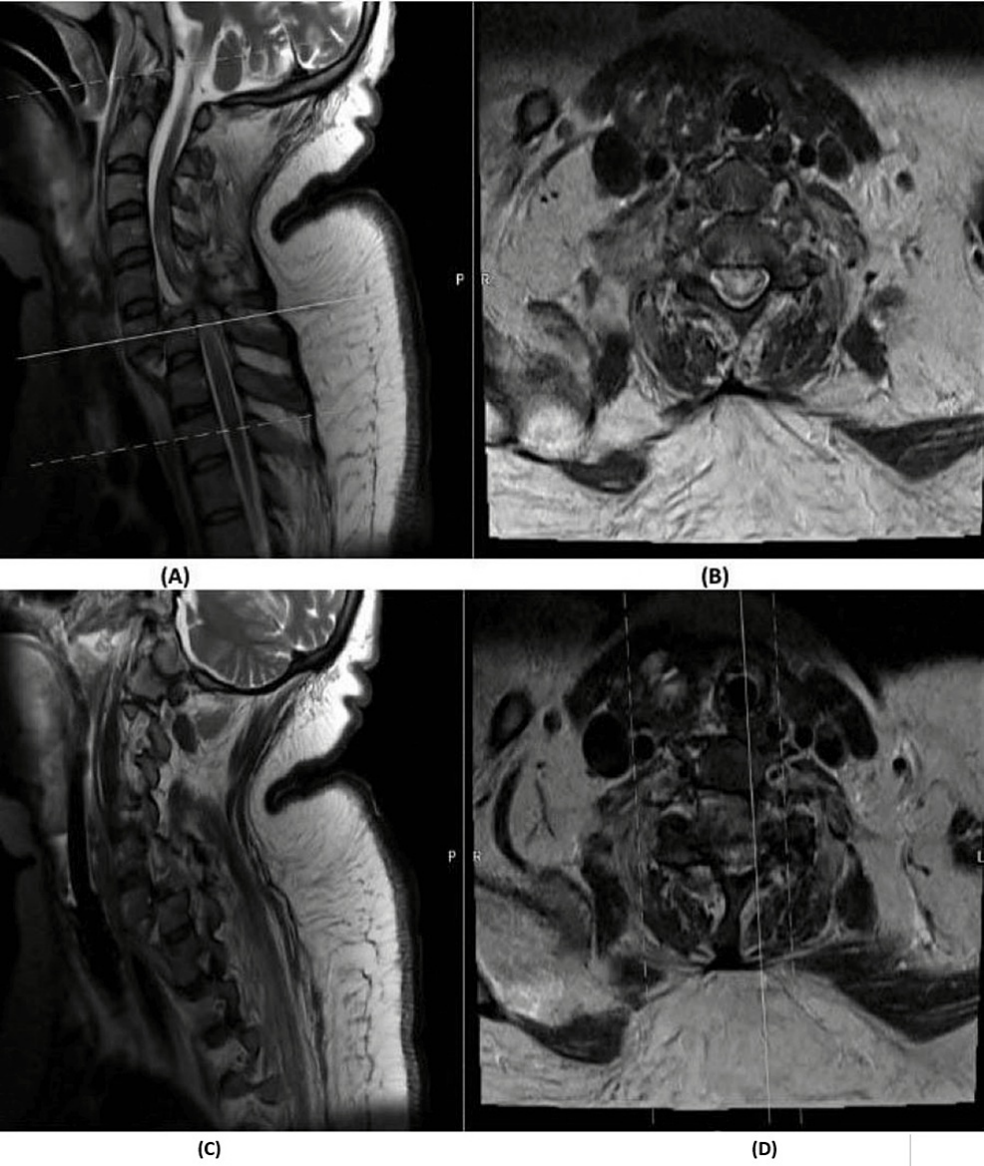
MRI of C6-C7 fracture dislocation (A,C): Sagittal spine MRI demonstrating a total fracture dislocation of C6–C7 with marked cord contusion. (B,D) Axial spine MRI of the corresponding level of C6–C7 vertebral body.
